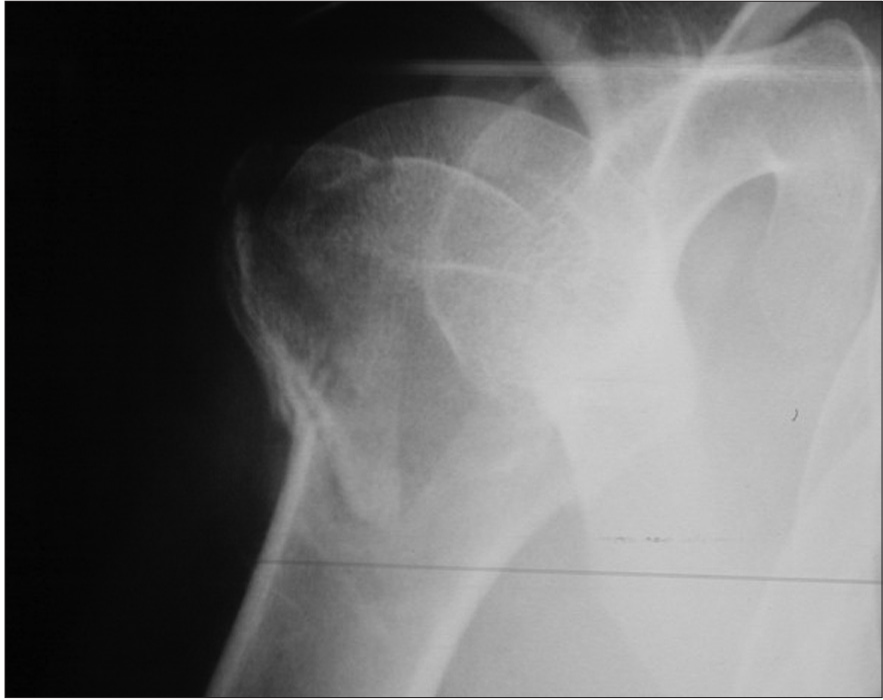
The radiographic appearance of the three-part fracture of the proximal humerus
